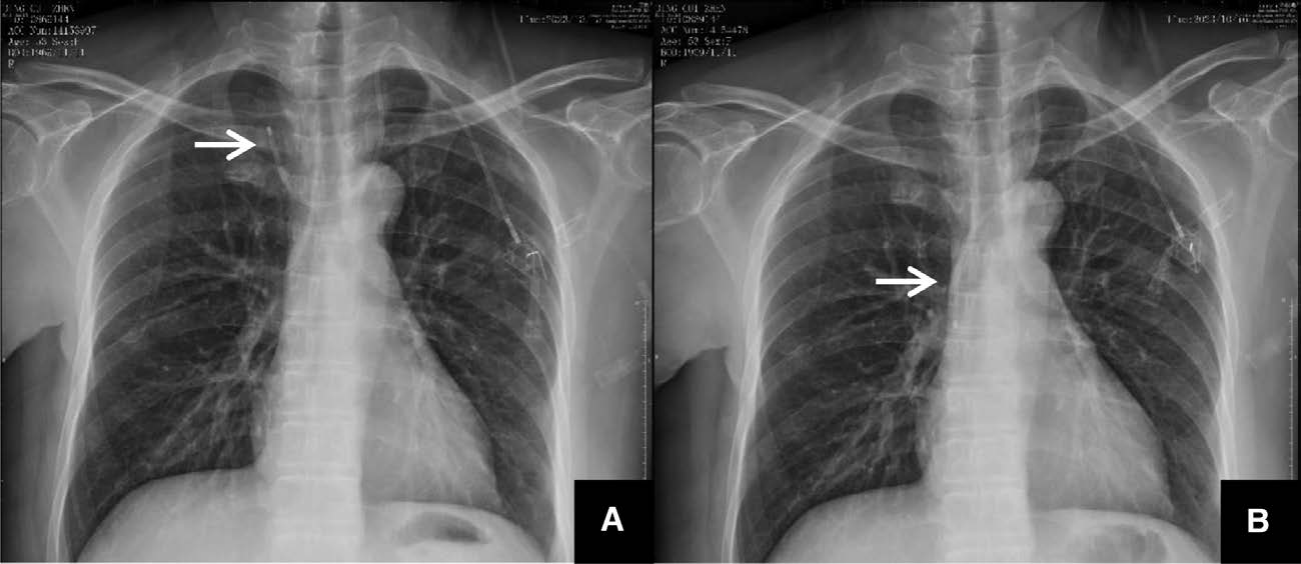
(A) Anteroposterior chest X-ray showing catheter tip malpositioned into the internal jugular vein. (B) Anteroposterior chest X-ray showing catheter tip repositioned.
